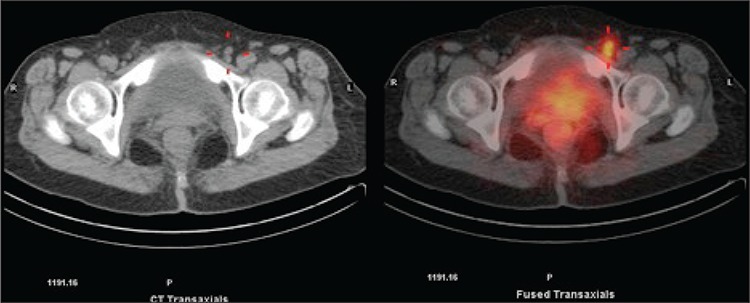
Hybrid SPECT/CT images of the pelvis showing focal tracer uptake in small left inguinal lymph node (Triangulation). Also noted is intense traceractivity in the vulva (site of injection)
